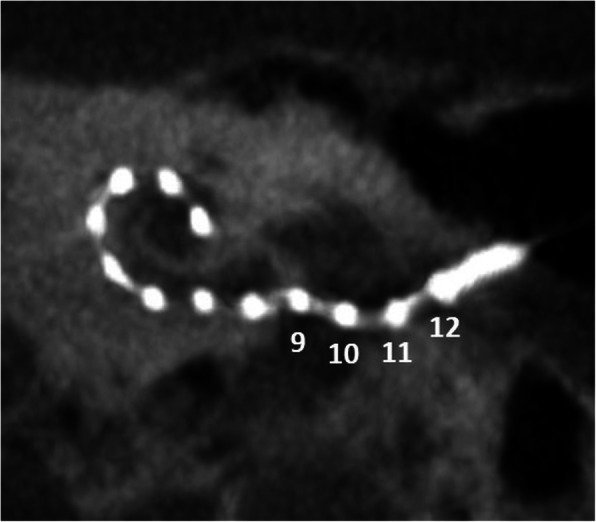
A 66-year-old female patient, with underinserted electrode. CBCT paraaxial maximum intensity projection image shows extracochlear location of electrode contacts 9 to 12
